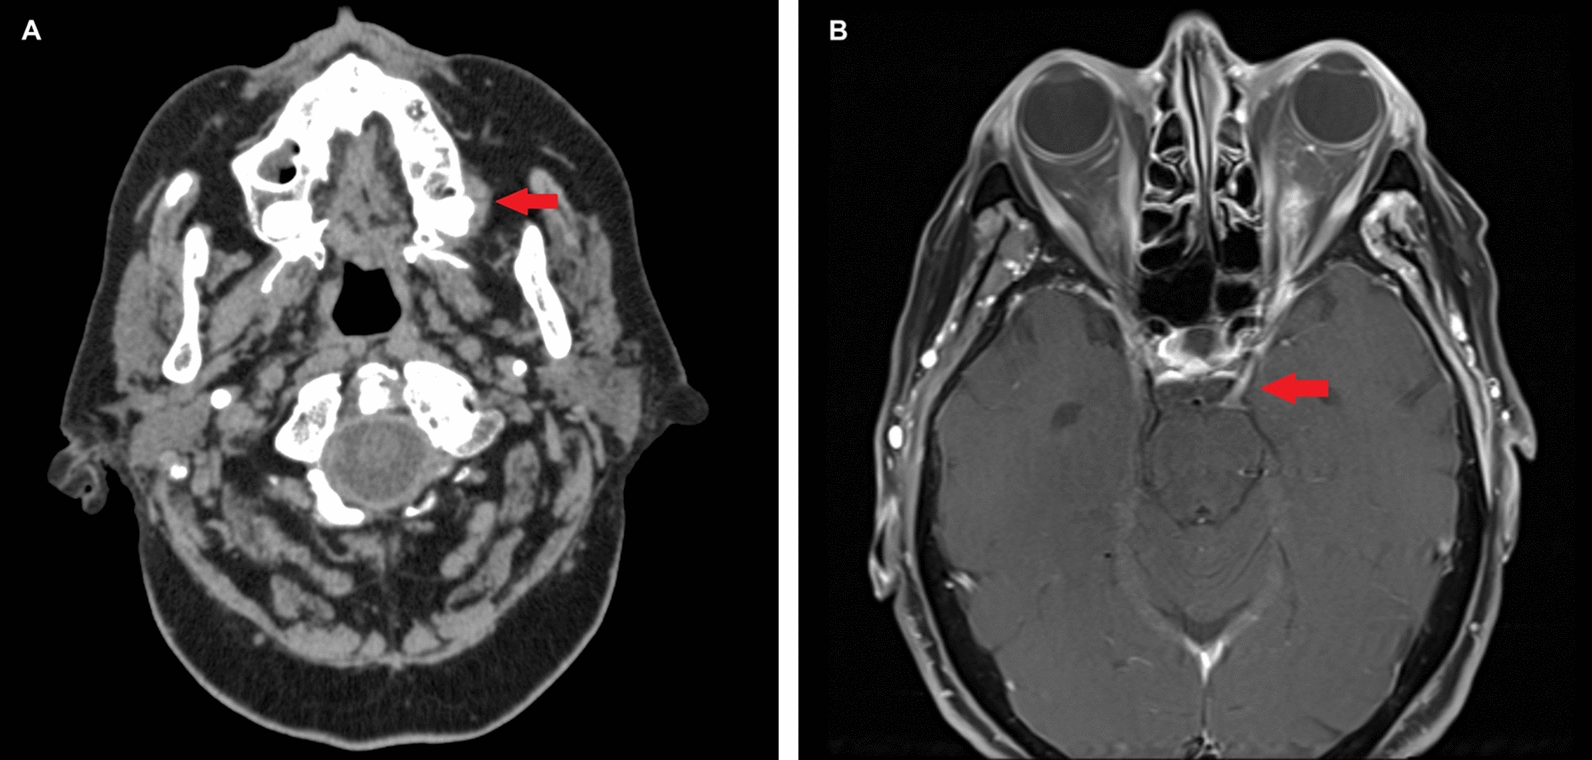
a Computed tomography scan: wisdom tooth with surrounding inflammation of the masticator space highlighted with red arrow. b Magnetic resonance imaging scan: exemplary oculomotor nerve with enhancement of contrast agent highlighted with red arrow
